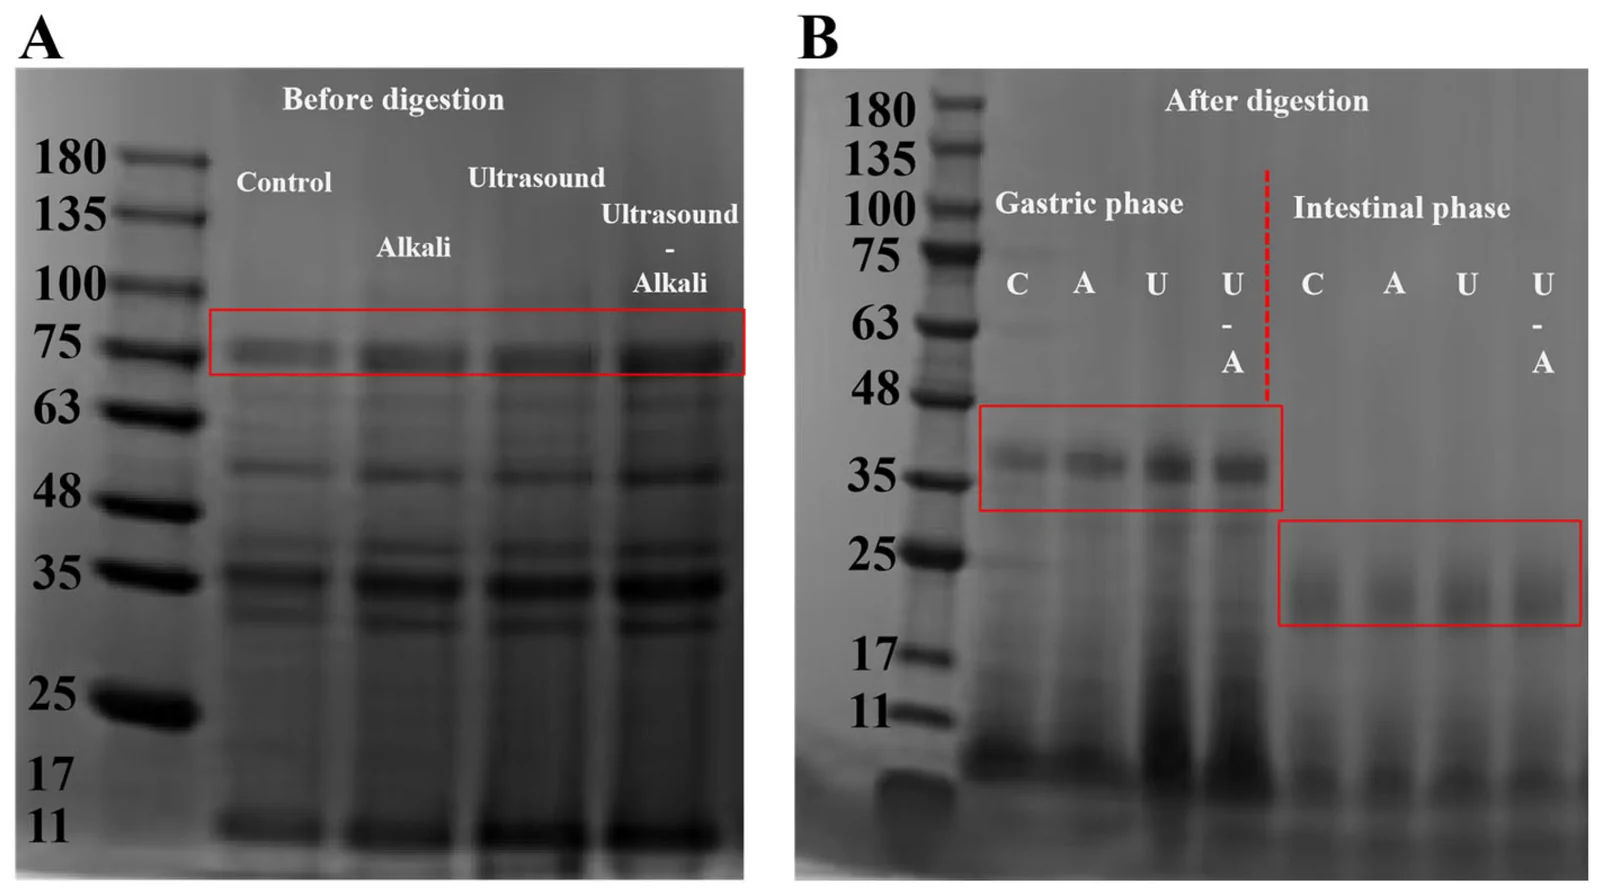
SDS-PAGE profiles of soybean flour before and after in vitro digestion. Before digestion (A), After digestion (gastric and intestinal phases) (B). Dashed lines separate different digestion stages. Abbreviations: C, Control; A, Alkali; U, Ultrasound; U-A, Ultrasound + Alkali. Molecular weight markers (kDa) are shown on the left.
